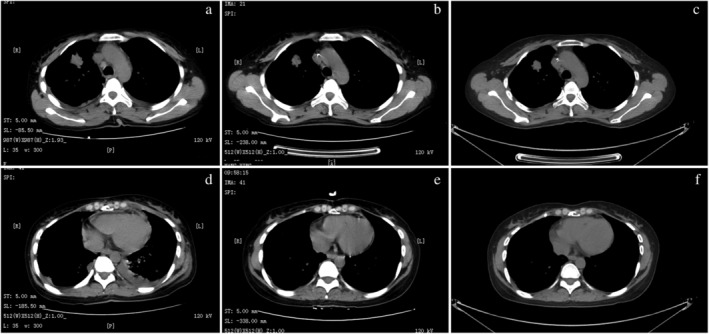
Computed tomography (CT) of the chest before and after four‐months treatment using chemotherapy combined with bevacizumab. Lung tumor regression is shown in (a) and (b); the pericardial effusion alteration is shown in (c) and (d).
